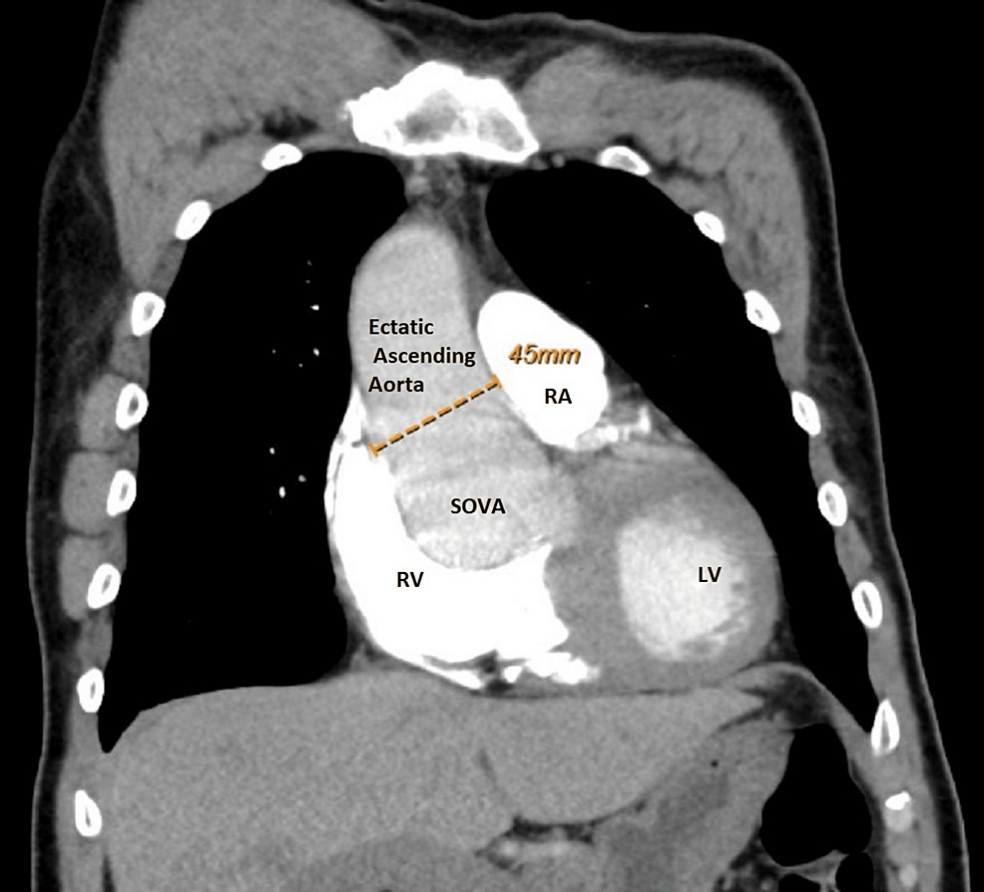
CT chest revealing ectatic ascending aorta to a diameter of 4.5 cm RA: Right Atrium; LV: Left Ventricle; RV: Right Ventricle; SOVA: SInus of Valsalva Aneurysm
